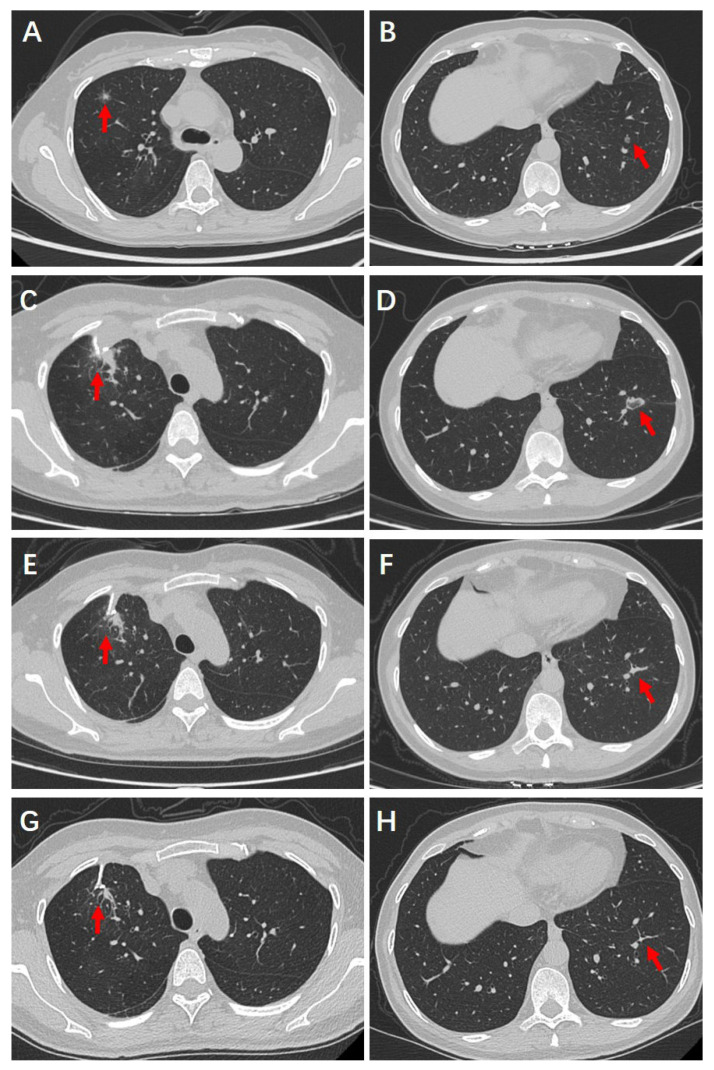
Pre-and postoperative High-Resolution Computed Tomography images of contralateral lesions managed by surgical resection and radiofrequency ablation. The primary part (right upper lobe)-solid nodule with spiculated margins underwent resection, while the contralateral lesion (left lower lobe) was ablated. Follow-up demonstrated progressive shrinkage and fibrosis of the lesions. (A) Resected lesion, preoperative. (B) Ablated lesion, preoperative. (C) One month after resection. (D) One month after ablation. (E) Three months after resection. (F) Three months after ablation. (G) Six months after resection. (H) Six months after ablation. The arrows indicate the location of the lesions and the changes observed during postoperative follow-up.
